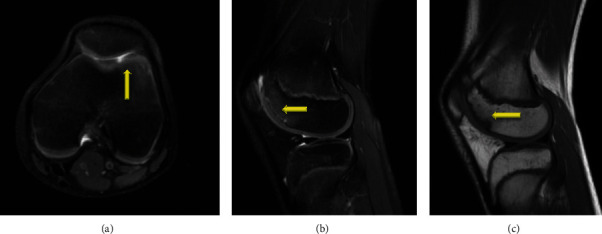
MRI evaluation of the knee showing stable trochlear osteochondritis dissecans (OCD) lesion on the (a) axial T2-weighted image, (b) sagittal T2-weighted image, and (c) sagittal T1-weighted image.
